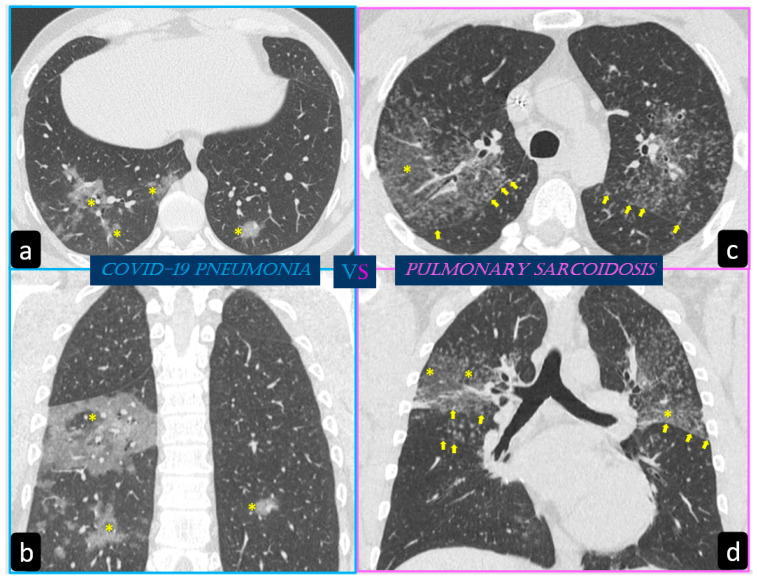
The images show a case of COVID-19 pneumonia (a,b) and a case of pulmonary sarcoidosis (c,d) both characterized by bilateral “ground glass opacities”. On the left side, axial (a) and coronal (b) HRCT scans show bilateral multiple ground glass opacities (yellow *) with peripheral distribution and lower lobe predilection (typical findings of COVID-19 pneumonia). On the right side, axial (c) and coronal (d) HRCT scans show (in addition to ground glass (GG) opacities) the typical perilymphatic distribution of micronodules also along the pulmonary fissures (yellow arrows) with upper lobes predilection (typical findings of pulmonary sarcoidosis).
